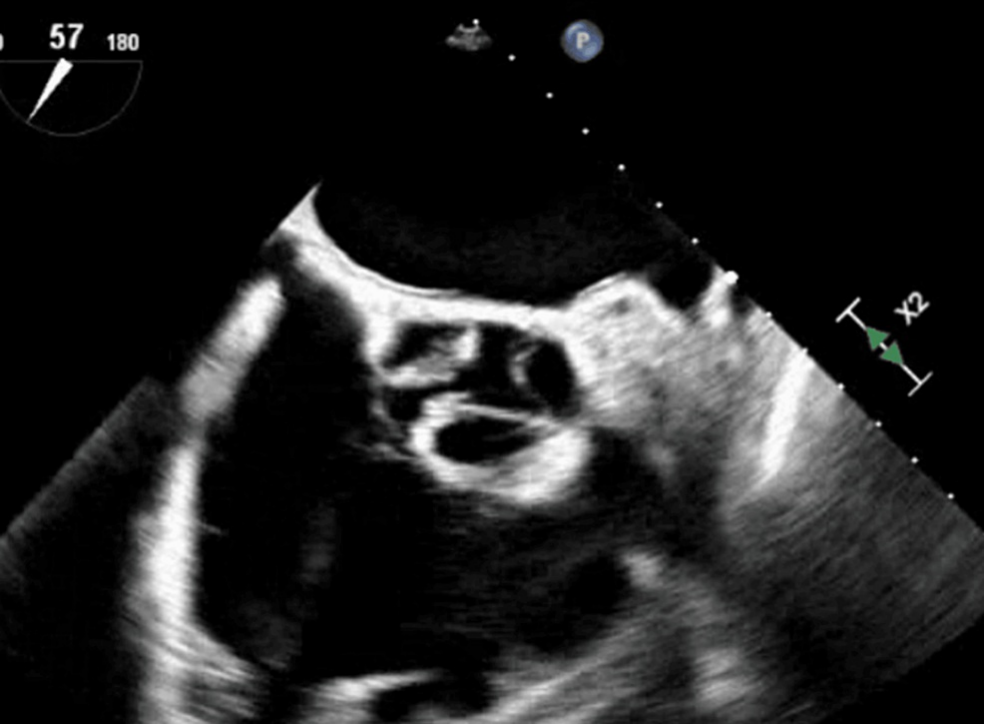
BAV seen on TEE with possible perforated left cusp and vegetation BAV, bicuspid aortic valve; TEE, transesophageal echocardiogram
